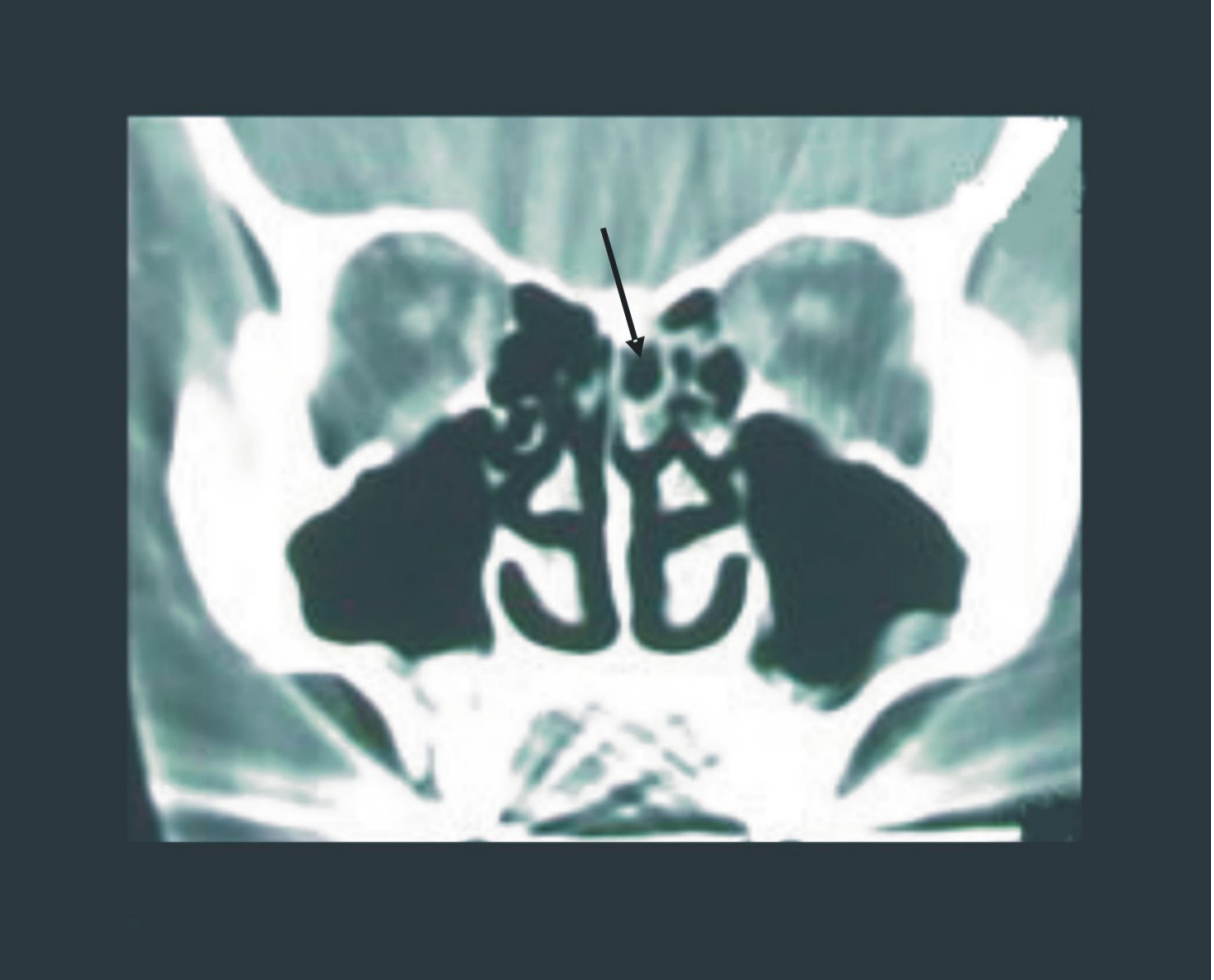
Coronal CT scan revealing pneumatization of the superior left turbinate (arrow).
